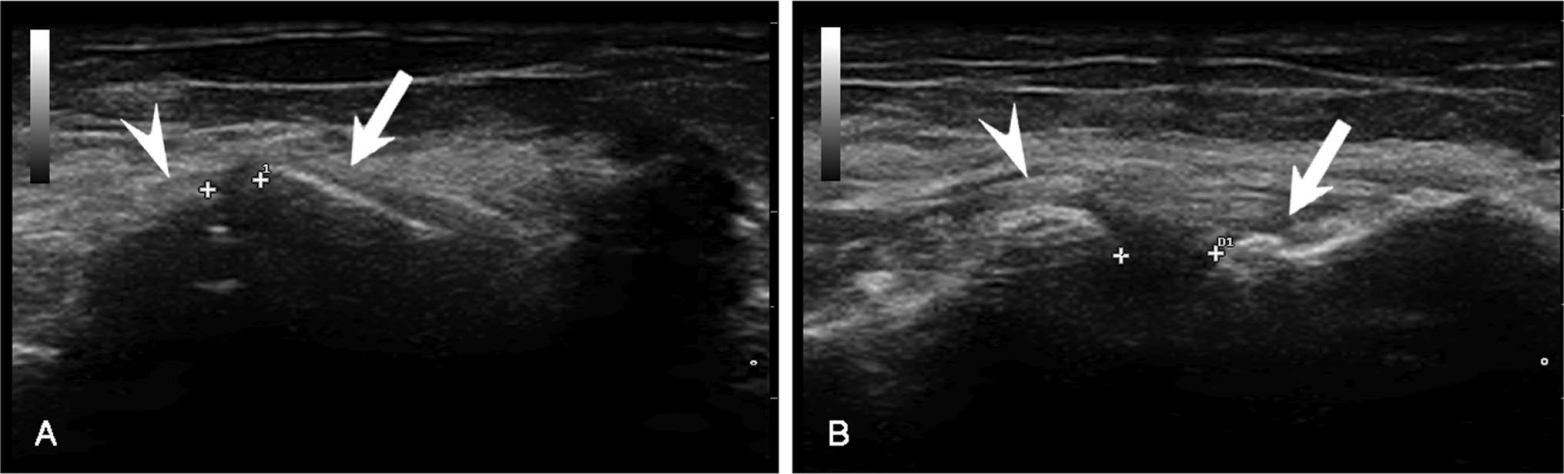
Dynamic ultrasound evaluation of the lateral compartment with 0.5 kg load applied to the hand before (A) and after (B) release of the anterior half of the common extensor origin. The arrow and the arrowhead indicate the lateral cortex of the radial head and the lateral cortex of the capitellum
